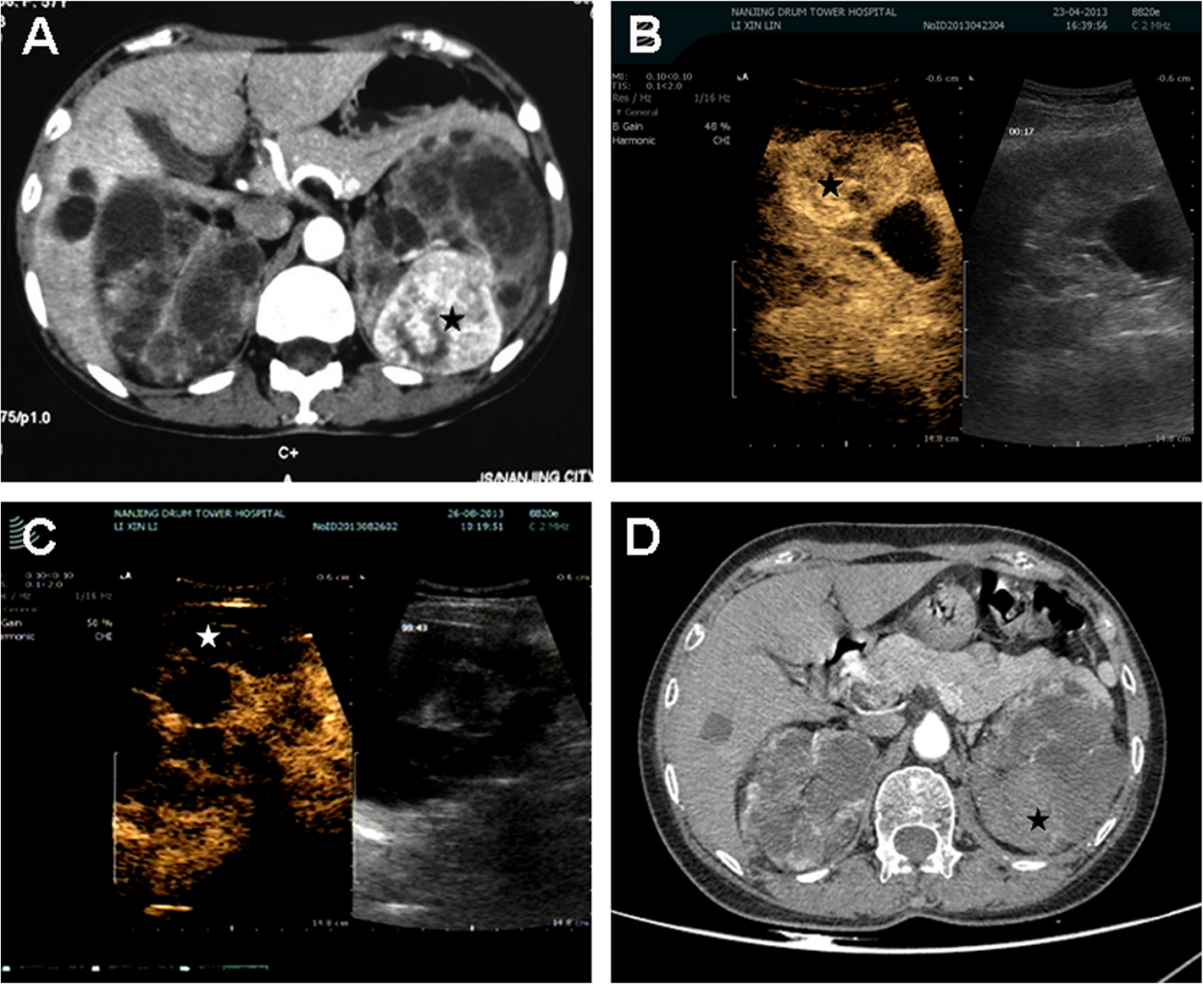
Percutaneous RFA was applied on a 57-year-old female ADPKD patient. The tumor was 5.2 cm in diameter and endophytically located in the posterior medial part of the left kidney. The tumor was completely ablated after two sessions of PRFA. a contrast-enhanced CT (arterial phase) obtained before PRFA showed enhancement of the tumor (*black star*). b CEUS shows the outline of the tumor (*black star*) before RFA. c CEUS was applied right after the final session of ablation and absence of enhancement was found in the tumor’s location (*black star*). d contrast-enhanced CT (arterial phase) obtained 1 year after final session of ablation shows absence of enhancement, indicating complete tumor necrosis (*black star*)
